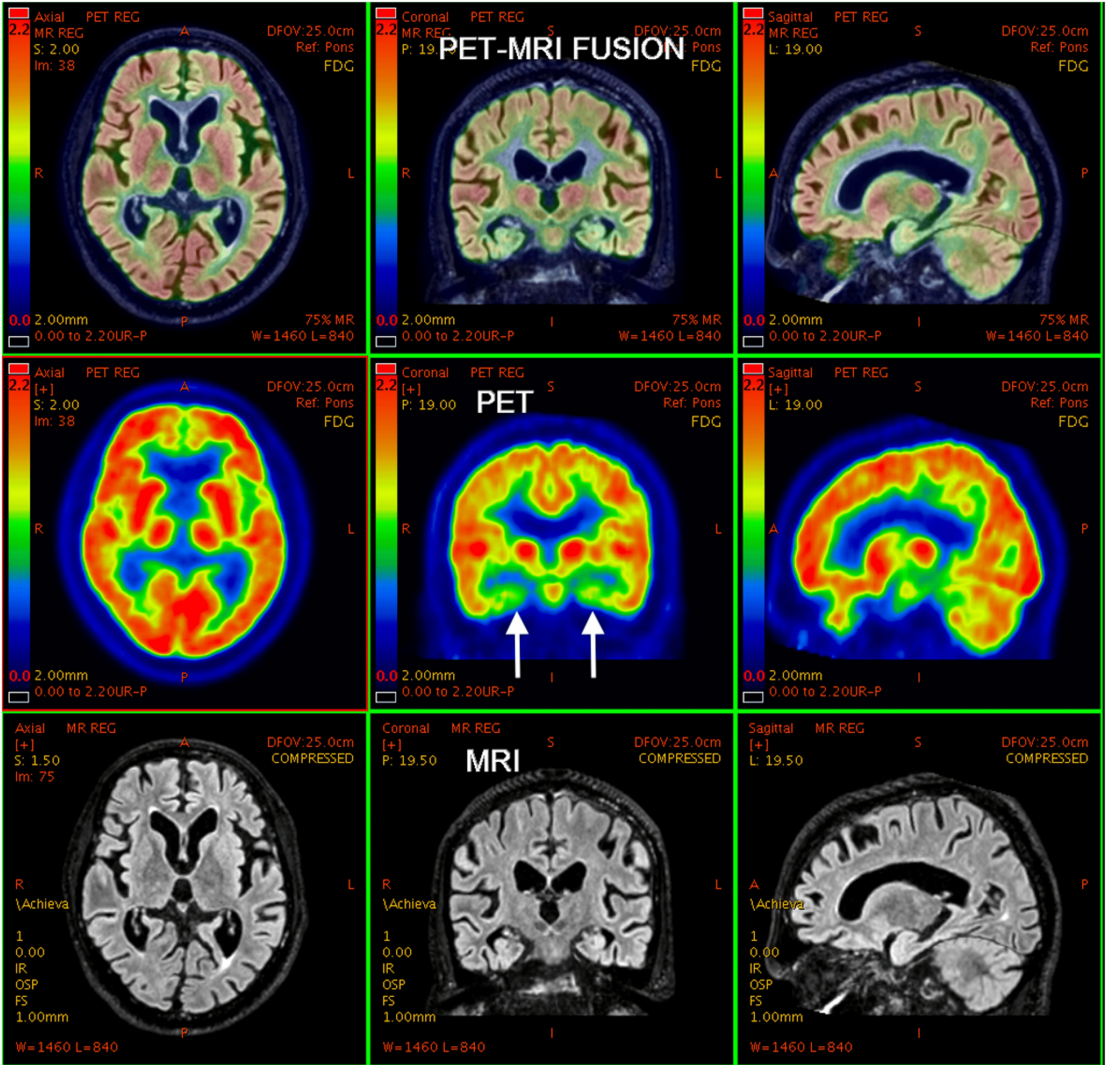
54-year-old male with progressive imbalance, forgetfulness, and dysarthria for one year. FDG uptake shows a homogeneous and symmetrical pattern in bilateral basal ganglia, frontal eye fields, posterior cingulate cortex, and visual cortex. Mild, relatively lower metabolic activity is observed in the medial temporal cortex. No areas of hypometabolism are noted. No metabolic alterations are seen. PET: positron emission tomography; MRI: magnetic resonance imaging; FDG: fluorodeoxyglucose
